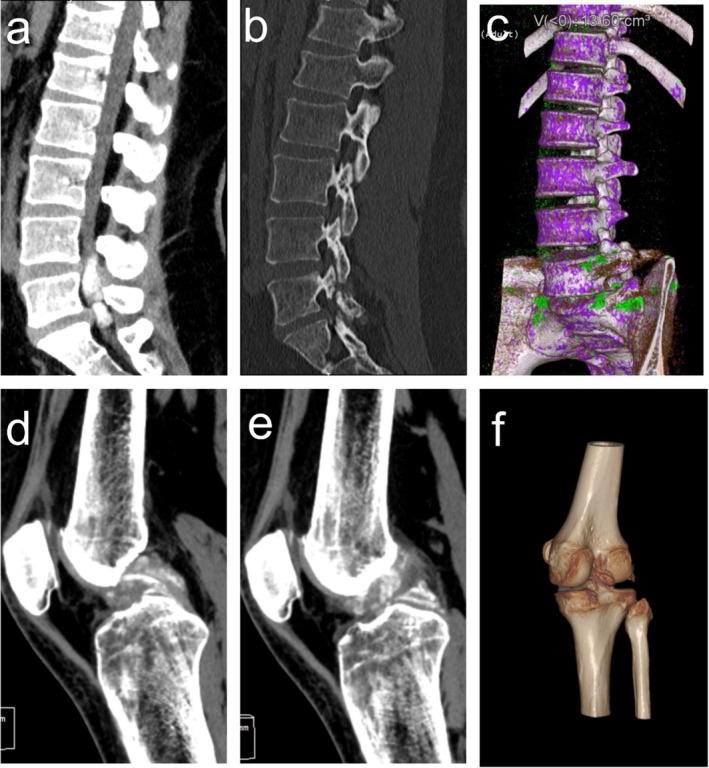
CT Scan of the Spine and knee. (a) CT image showing the presence of gouty granulomas within the spinal canal at the L5‐S1 level. (b) CT scan showing the isthmic fracture and the disc herniation at the L5‐S1 level. Notable findings include changes in bone density and the presence of gouty stones. (c) Dual‐Energy CT (DECT) showing the presence of gouty stones. (d–f) (DECT) a large number of gouty stones in the knee joints.
